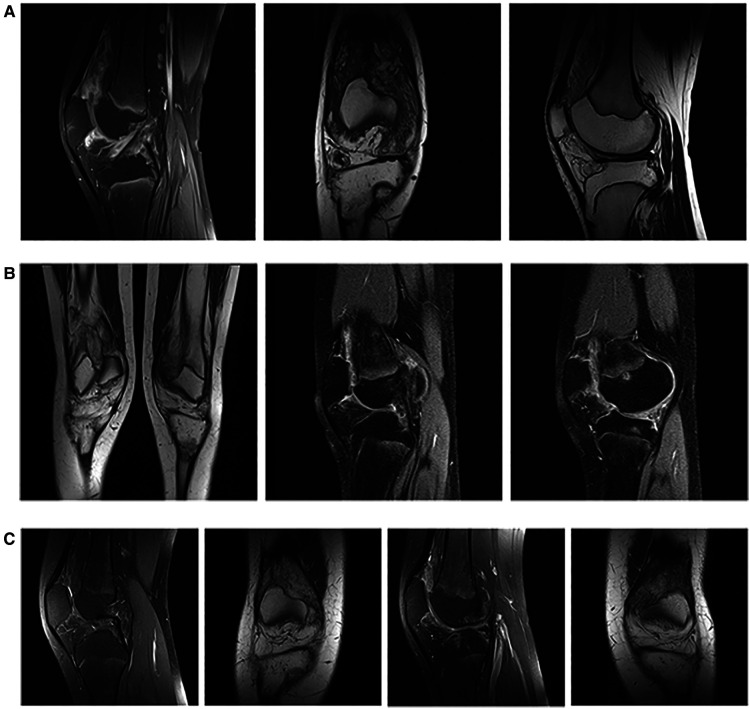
Imaging examinations. (A) Initial MRI scanning of the right knee [MRI Proton Density with Fat Saturation (PDFS), sagittal plane; MRI T1 weighted image, coronal plane; MRI T1 weighted image, sagittal plane] showed the extended intra-articular synovial proliferation with fat inclusions combined with multiple marginal bony erosion of the proximal tibia, both femoral condyles and patella along with altered morphology of both menisci. (B) Control MRI of both knees (MRI T1 weighted image, coronal plane; MRI Proton Density with Fat Saturation (PDFS), sagittal plane, right; MRI Proton Density with Fat Saturation (PDFS), sagittal plane, left) performed after 2 years of MTX therapy with almost the same, bilateral lesions as one on the initial MRI of the right knee. (C) Second MRI control of both knees [MRI Proton Density with Fat Saturation (PDFS), sagittal/coronal plane, right/left] with almost complete regression of the synovial proliferation in the right, and partial regression in the left knee after 6 months of TNF inhibitor therapy.
